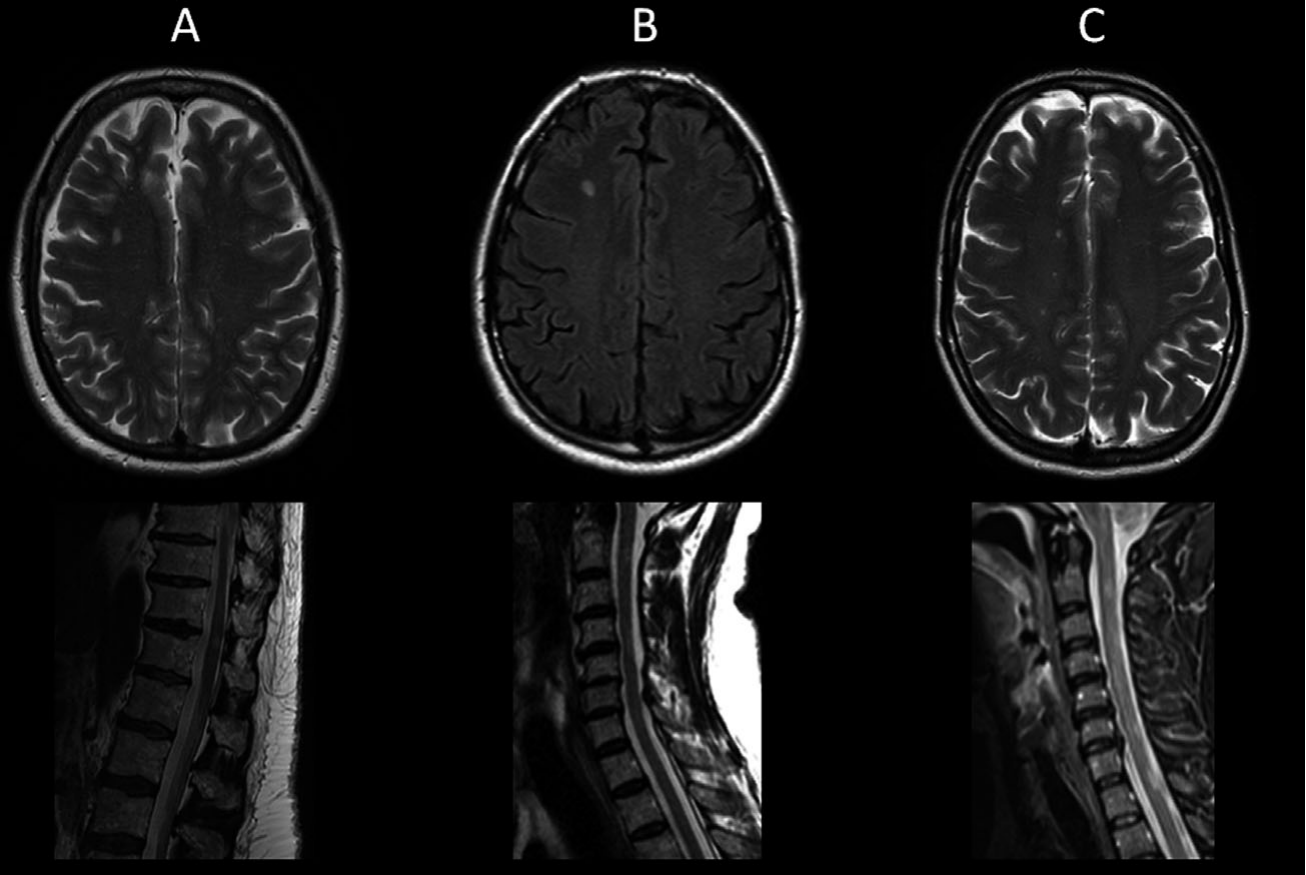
Brain and spinal cord MRI abnormalities in three patients with a multiple sclerosis‐like disorder and *OPA1* mutations. Multiple foci of T2 hyperintensity were observed in the periventricular and pericallosal white matter regions. Discreet areas of signal abnormalities were also present in the cervical spinal cord, none of which were longitudinally extensive. Taken together, these neuroradiological findings met the MRI diagnostic criteria for multiple sclerosis. Panel A is patient A, panel B is patient B, and panel C is patient C. Upper panel A = axial T2‐weighted imaging, lower panel A = sagittal T2‐weighted imaging. Upper panel B = axial T1‐weighted imaging, lower panel B = sagittal T2‐weighted imaging. Upper panel C = axial T2‐weighted imaging, lower panel C = sagittal T2‐weighted imaging.
